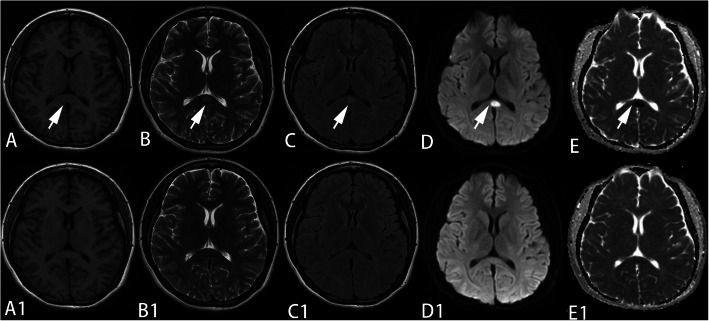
The MRI findings of case 7. It showed oval-shaped center on SCC characterized by hypo-intensity on T1-FLAIR (A), hyper-intensity on T2WI (B), T2-FLAIR (C) and DWI (D) and low ADC value (E). The lesions were resolved in the follow-up (A1-E1)
